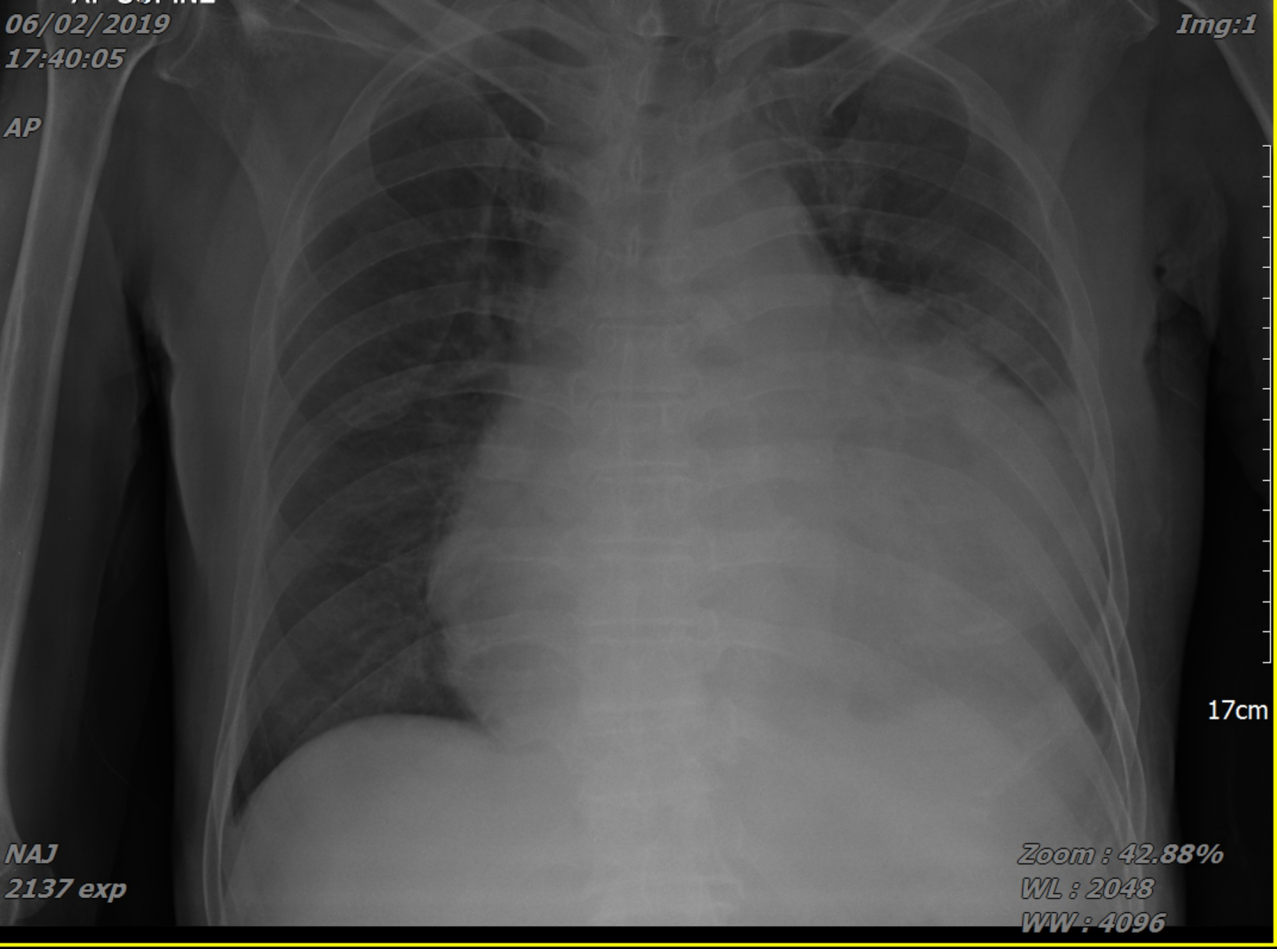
Chest radiograph upon admission showing cardiomegaly, pulmonary congestion and bilateral pleural effusion.
